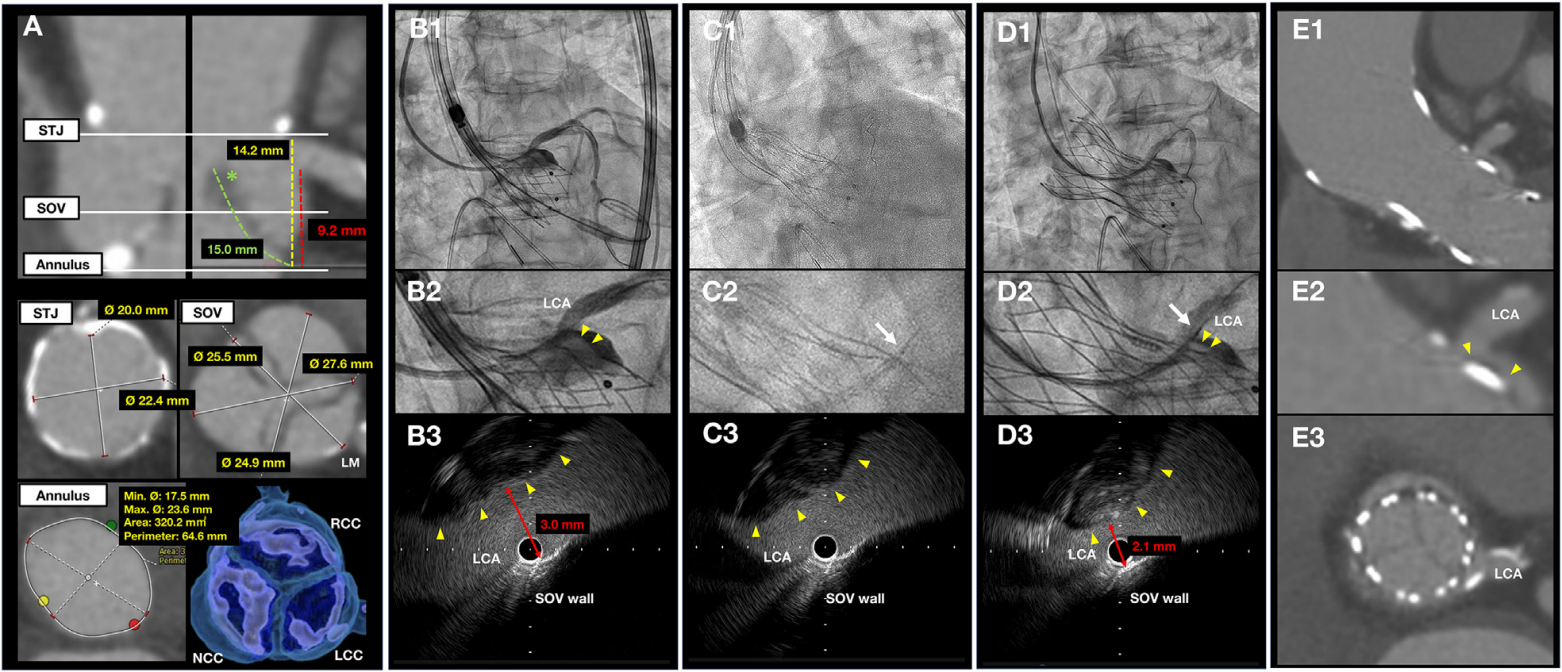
Real-Time Leaflet Monitoring Using IVUS During Transcatheter Aortic Valve Implantation (A) Preprocedural computed tomography showing a long LCC leaflet (green asterisk) and low LCA height. (B) At the point of no recapture, (C) during the THV release, and (D) after the THV release, IVUS images enabled clear visualization of a positional relationship between the tilted-up leaflet (yellow arrowheads) and the LCA ostium. White arrows indicate IVUS catheter placement in the LCA ostium. (E) Postprocedural computed tomography showing a patent LCA ostium. IVUS = intravascular ultrasound; LCA = left coronary artery; LCC = left coronary cusp; NCC = noncoronary cusp; RCC = right coronary cusp; SOV = sinus of Valsalva; STJ = sinotubular junction; THV = transcatheter heart valve.
